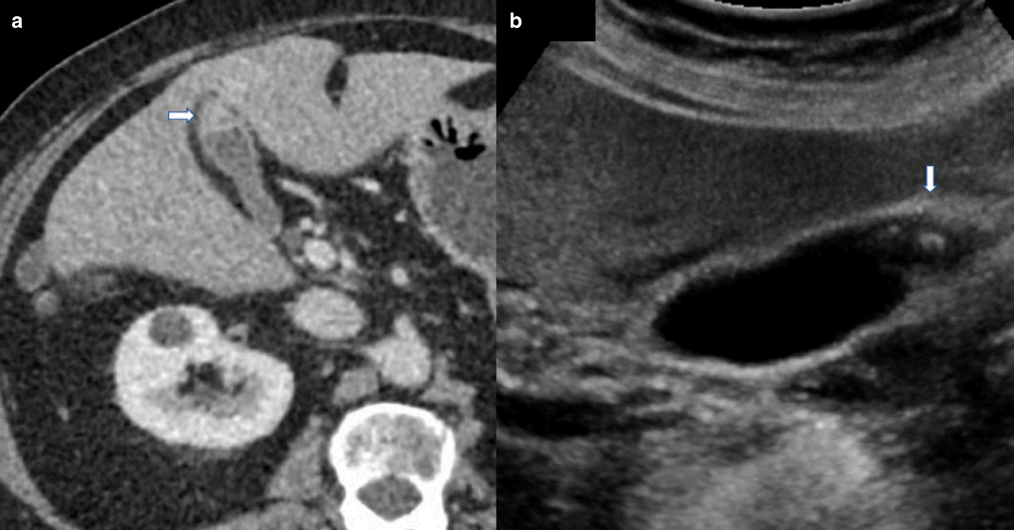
(a) Axial contrast-enhanced CT showing discrete focal thickening of the gallbladder fundus with normal mucosal enhancement, typical of fundal adenomyomatosis. (b) Greyscale ultrasound confirms nodular, hyperechoic foci studding the wall of the gallbladder causing reverberation artefact of the cholesterol crystals trapped in the mucosal sinuses—the classical “comet-tail” artefact (white arrow). Comet tail artefact can also be a feature of pseudopolyps and cholesterol polyps.
